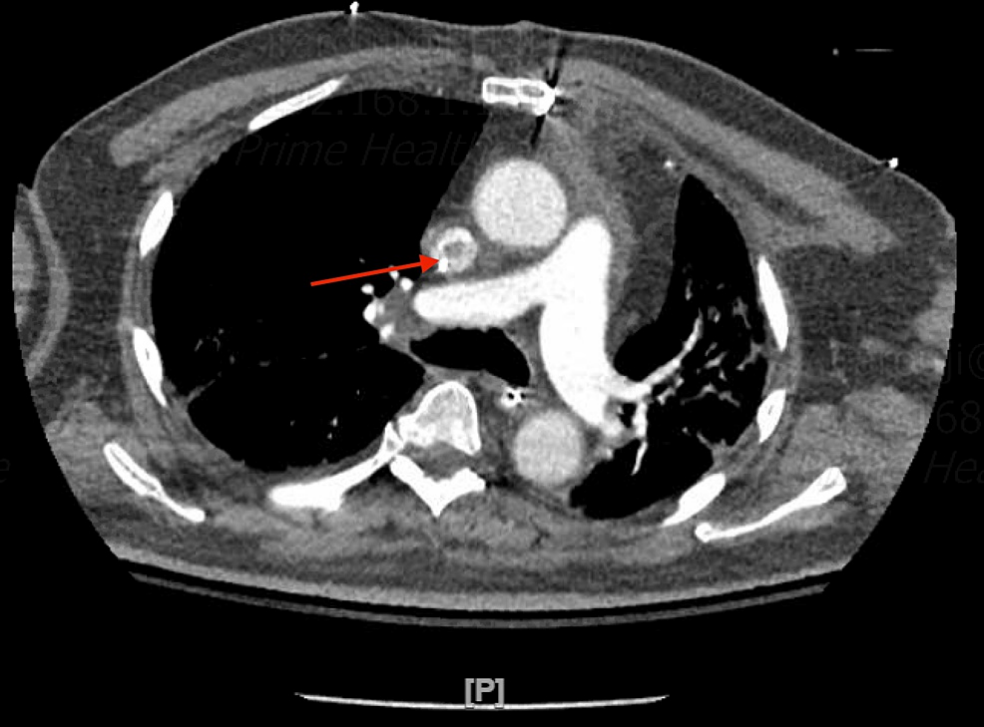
CTA of the chest revealed SVC thrombosis. CTA: computerized tomographic angiogram; SVC: superior vena cava
